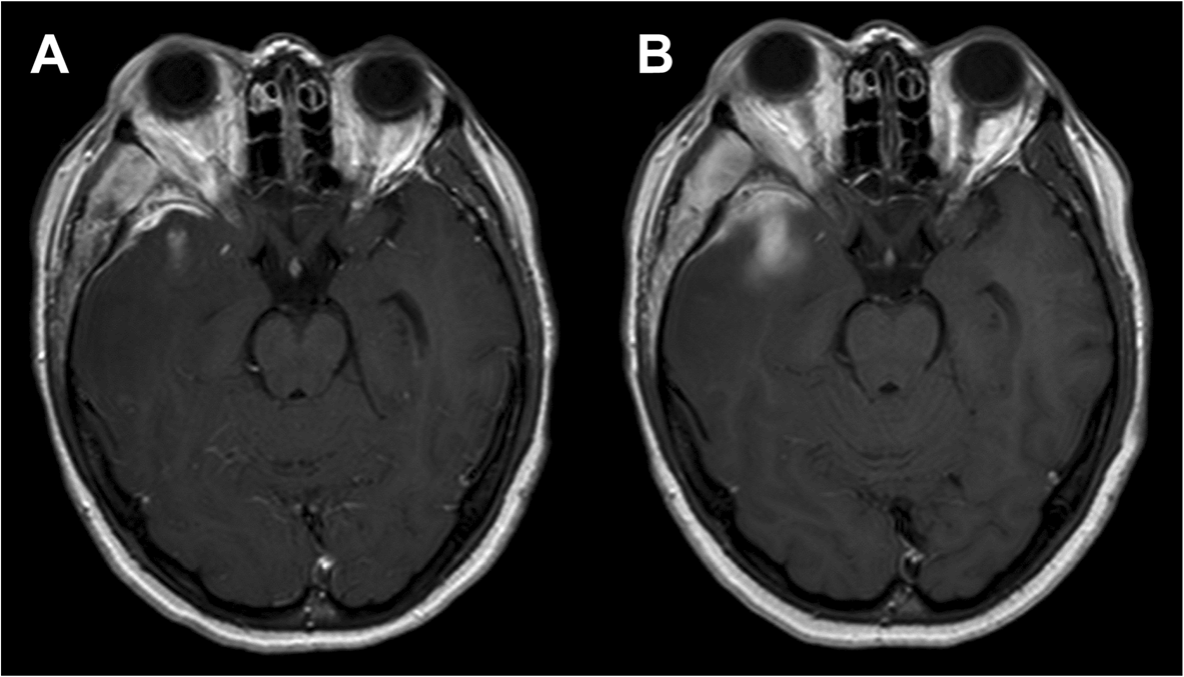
Early and delayed intracranial enhancement in the acute phase. Axial CE T1w MR images before treatment at the level of the middle cranial fossa obtained 5 min (a) and 1 h (b) after iv administration of Gadolinium. Substantial thickening and intense enhancement of the dura mater adjacent to the right sphenoid wing can be appreciated (a), as well as involvement of the sub-cortical white matter of the temporal pole, that increases in the delayed phase (b). The temporal muscle also appears markedly enlarged and intensely enhancing compared to the contralateral one
